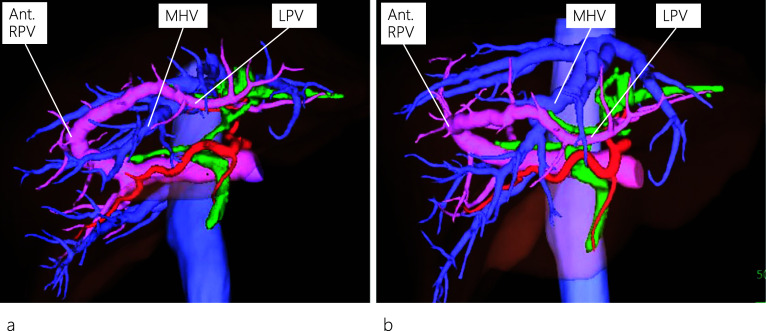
Three-dimensional reconstruction of the enhanced CT images. The left hepatic artery and bile duct are separated from the left portal vein (LPV) instead of being located in the left Glisson’s sheath as viewed from a left anterior oblique position (a). LPV, viewed from a neutral position, runs through the ventral side of the middle hepatic vein (MHV) (b). MHV: middle hepatic vein; Ant. RPV: anterior right portal vein; LPV: left portal vein
